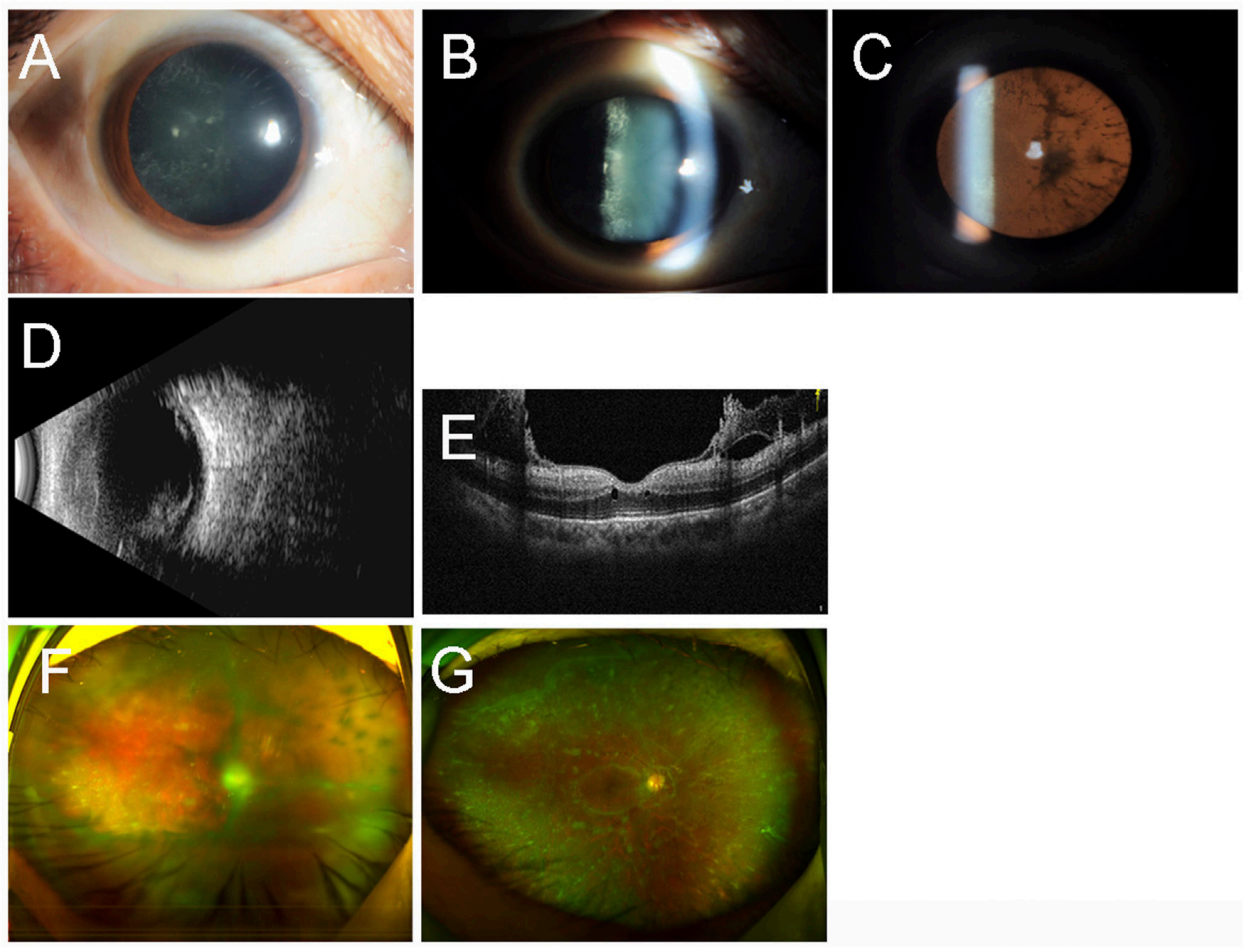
Ocular imaging findings of the right eye of the proband (III-13). Slit-lamp examination (A–C) revealed amyloid deposition in the posterior lens capsule. B-mode ultrasonography (B) showed mass echoes in the vitreous cavity. Ocular coherence tomography (E) confirmed the glasswool-like posterior vitreous cortex attached to the retina. Ultra-wide-field scanning laser ophthalmoscopy revealed vitreous opacities before the surgery (F) and one month after the surgery (G).
